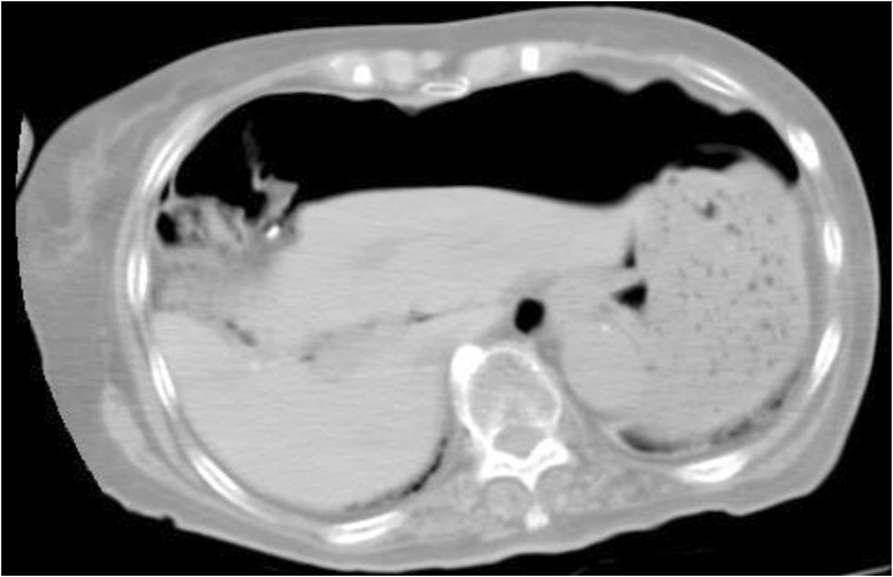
Abdominal computed tomography. Computed tomography findings show a massive pneumoperitoneum localized to the upper abdomen without collection of intra-abdominal fluid, pneumoretroperitoneum, or subcutaneous emphysema
